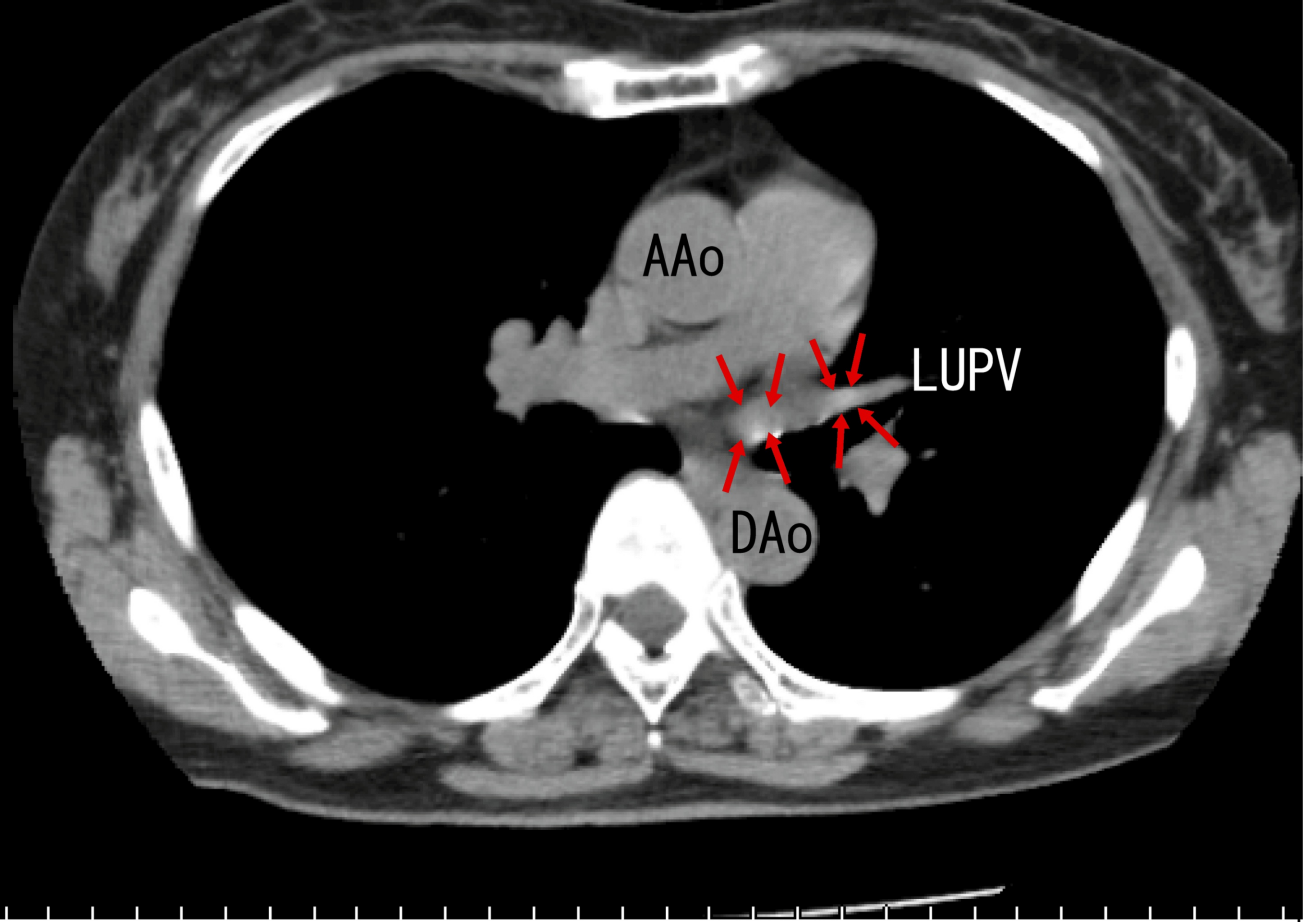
Axial plain CT images Axial images depict calcifications in the LUPV as two white areas (arrows). AAo: ascending aorta, DAo: descending aorta, LUPV: left upper pulmonary vein
